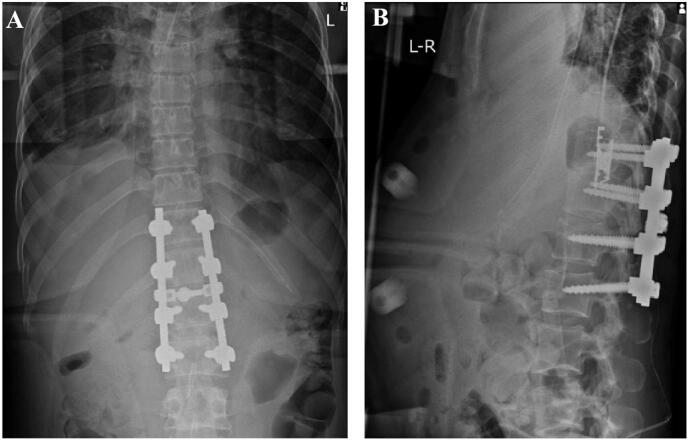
The postoperative X-ray images demonstrated good reduction on both anteroposterior (A) and lateral views (B).
